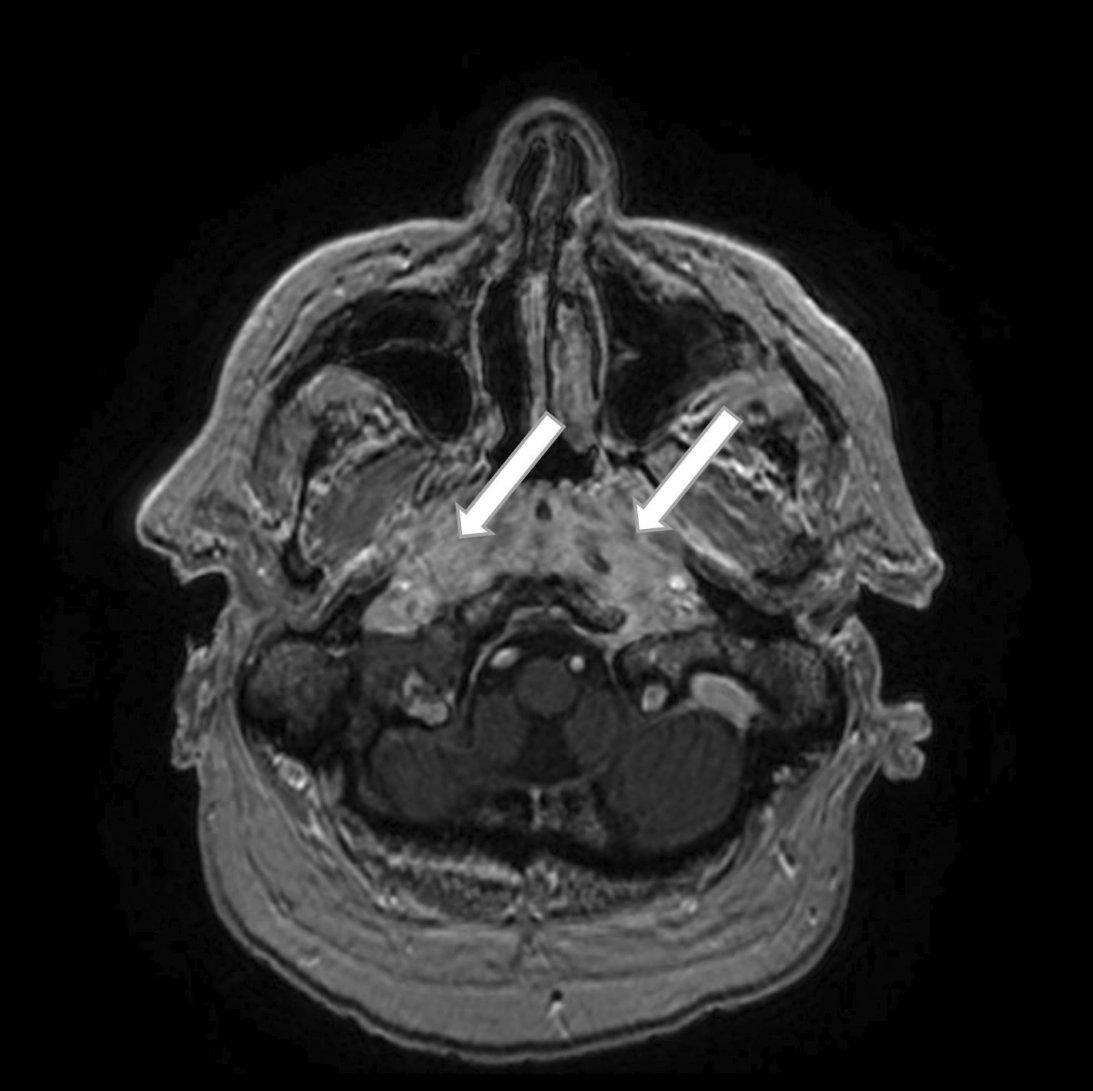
Axial fat‐sat MRI scan showing a bilateral contrast‐enhanced lesion in the rhinopharynx (simple arrows).
